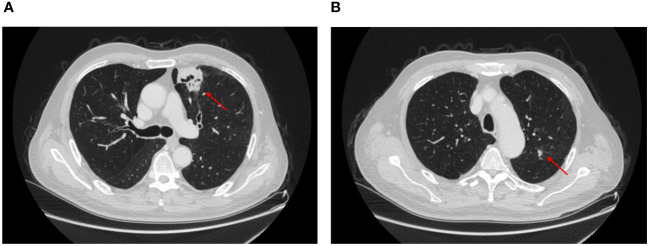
CT showed the location of the pulmonary nodule in the left upper lung. (A) squamous cell carcinoma, (B) adenocarcinoma.
